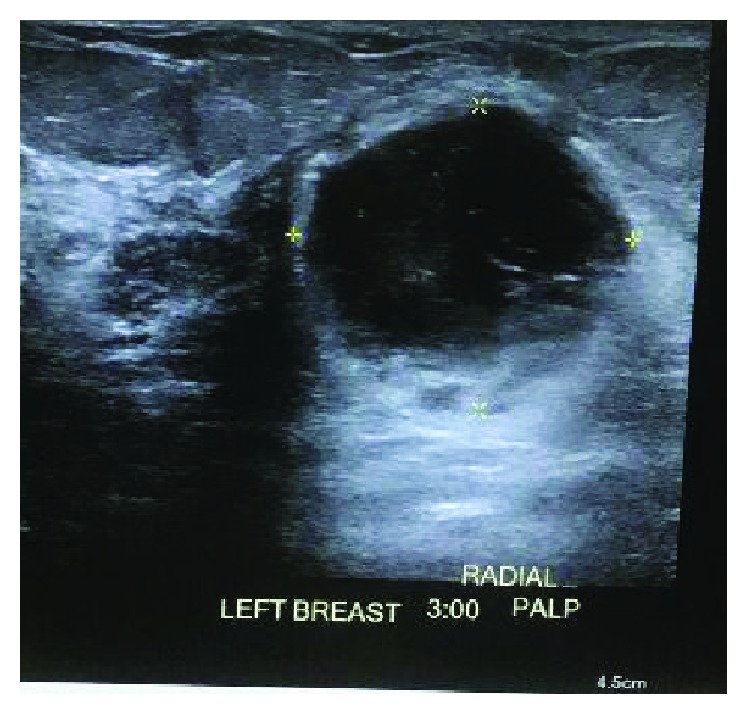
Left breast sonogram showing a cystic mass.
